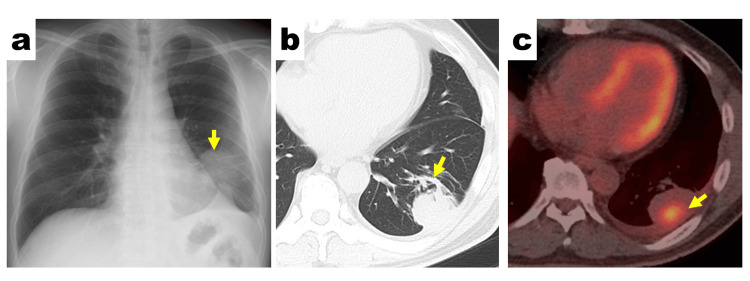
Preoperative radiological findings a. Chest radiograph showing an abnormal shadow in the left lower lung field (yellow arrow). b. Chest CT image revealing a 45-mm solid tumor with a comet tail sign (yellow arrow) in the left lower lung lobe (S9). c. Positron emission tomography with CT showing localized accumulation of ^18^F-FDG (yellow arrow). The maximum standardized uptake value of the lesion was 6.5. ^18^F-FDG: ^18^F-fluorodeoxyglucose; CT: computed tomography
